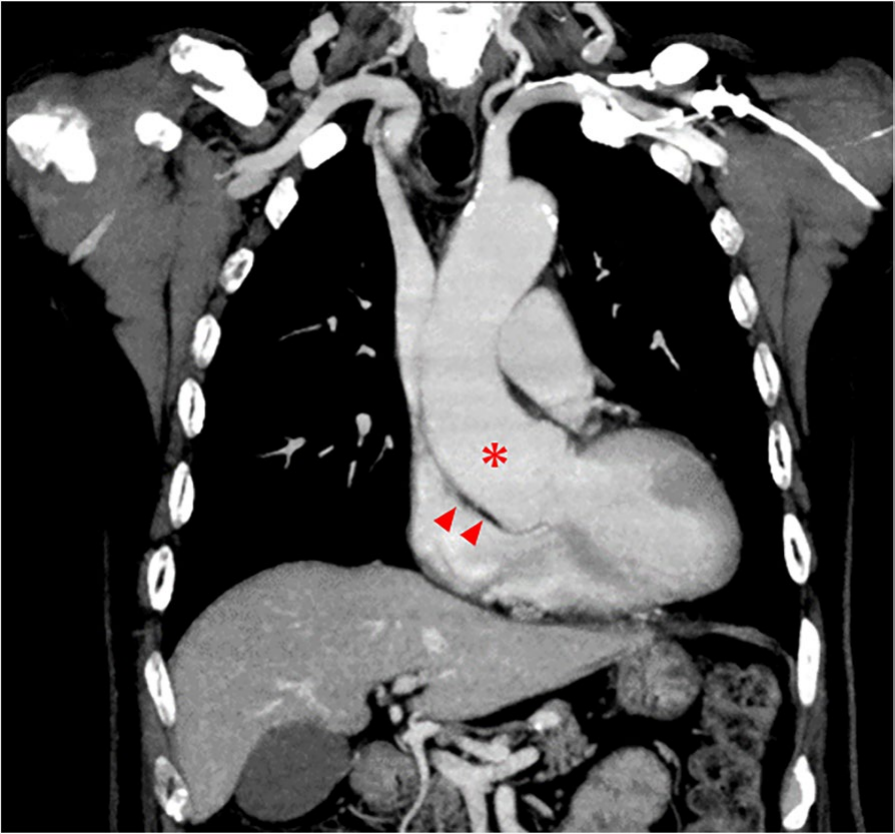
Coronal computed tomography section of the heart. A remarkably enlarged aortic root (asterisk) compressed the right atrium (arrowhead)
